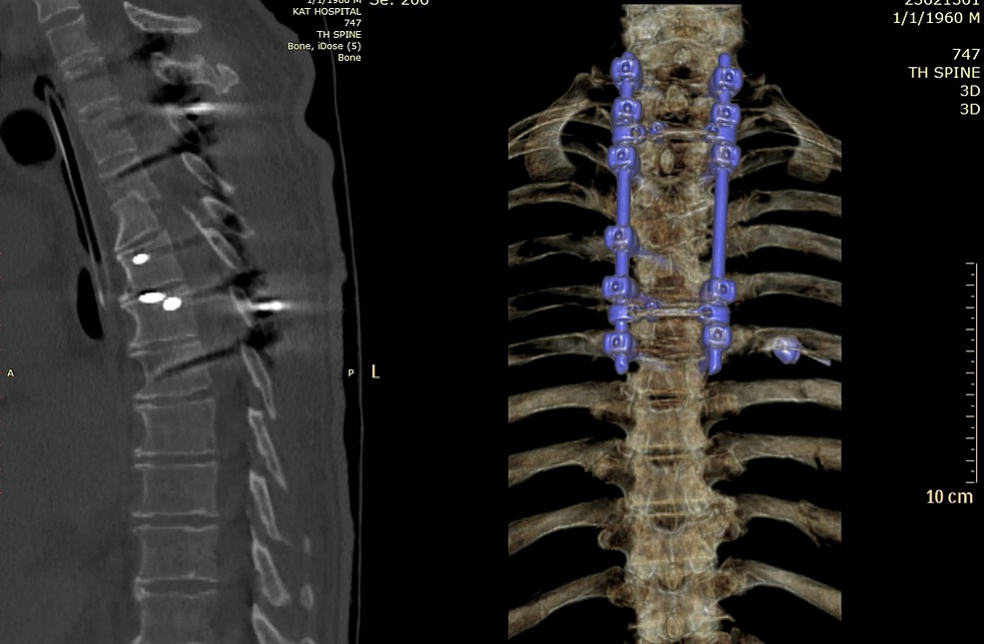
Postoperative CT scan Sufficient alignment of thoracic spine 10 days postoperatively.
